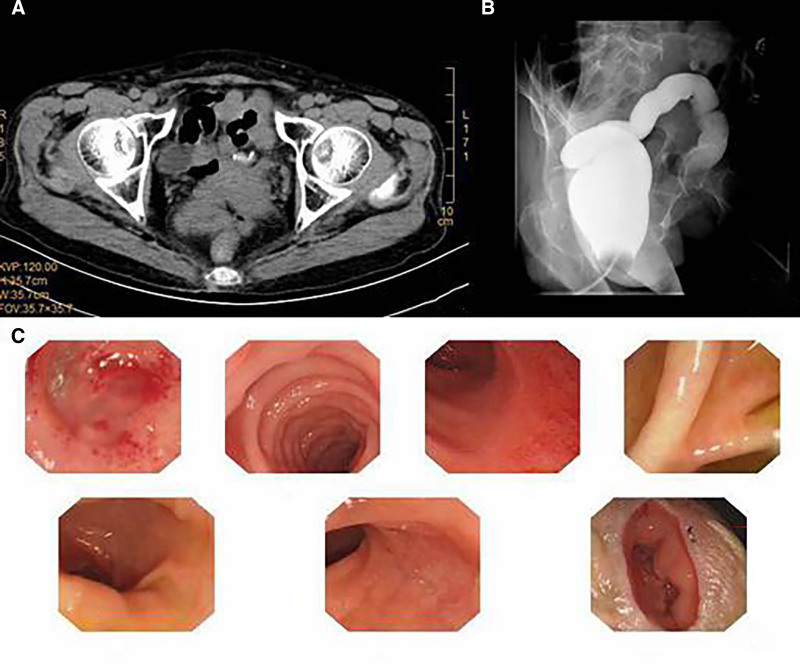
(A) CT demonstrating surgical clips at the colorectal anastomosis site. (B) Barium enema imaging demonstrated barium passage through the anastomotic fistula into the sigmoid colon. The sigmoid colon appeared dilated, without evidence of stricture, obstruction, or filling defects. (C) Colonoscopy revealed normal mucosa from the descending colon to the ileocecal valve. Localized mild congestion and edema were observed in the mucosa of the rectum and residual sigmoid colon. A single exposed suture staple was visible at the left lower quadrant abdominal stoma. CT = computed tomography.
